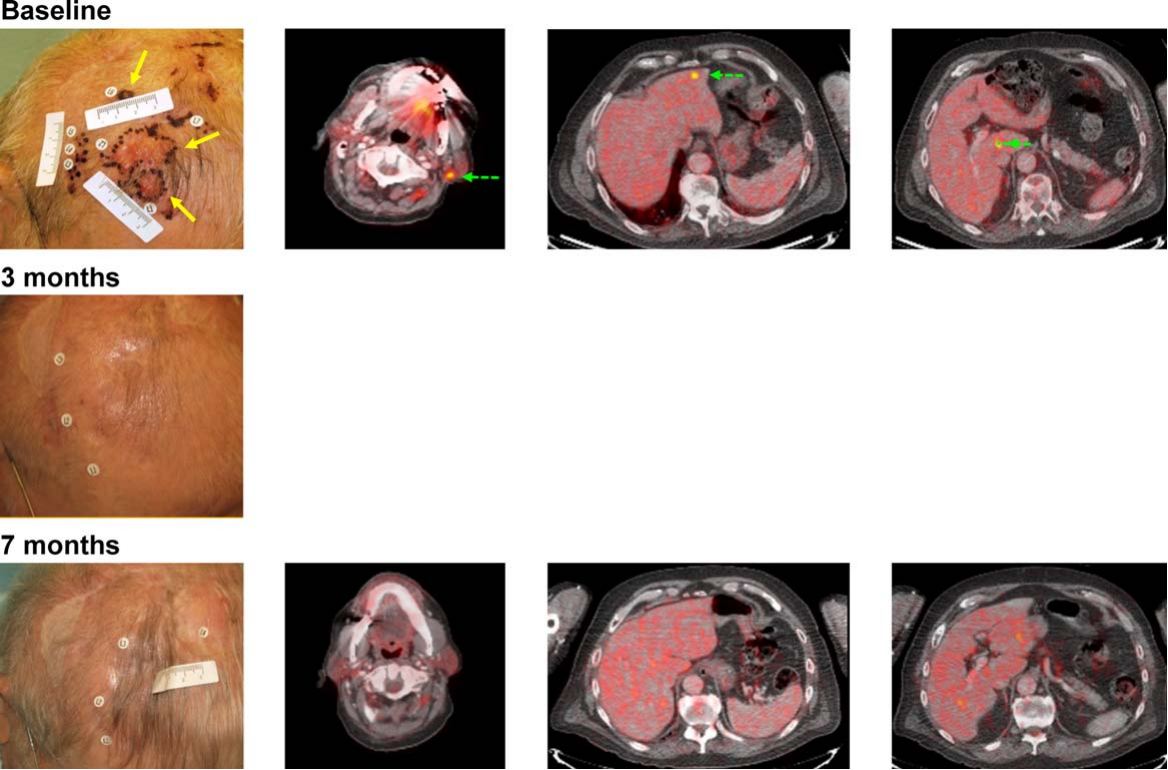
(A) Representative images from a patient with melanoma of the scalp with metastasis to cervical lymph nodes and liver (stage IVM1c). The patient was diagnosed 2 years before enrollment in OPTiM and had 2 surgeries: one at diagnosis, and another 1 year after recurrence. Top row: injection sites shown in yellow arrows at baseline (left panel). Uninjected sites are shown with green dashed arrows. Black dots mark tumor lesions. Sites included 1 fluorodeoxyglucose (FDG)‐avid left upper level V cervical lymph node (center left panel) and 2 FDG‐avid liver lesions (center right and right panels). Middle row: injections were stopped after complete resolution of scalp lesions after cycle 2 (1 cycle = 2 injections of talimogene laherparepvec). Bottom row: Complete resolution of cervical and liver tumors was documented by FDG‐PET CT at cycle 7. Patient was in complete response until the end of the trial, duration of response (complete response) was approximately 17 months.
